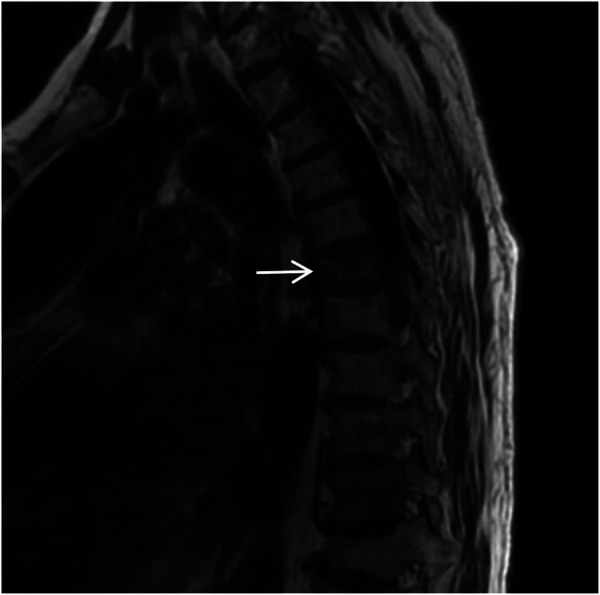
Sagittal T1-weighted MRI of the thoracic spine shows a flattened T6 (white arrow) vertebral body with patchy low signal within the vertebral body.
